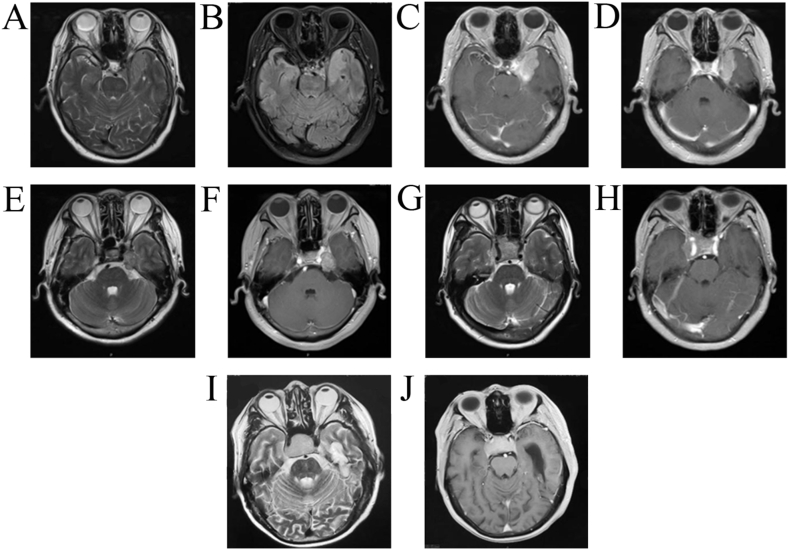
Imaging characteristics of cavernous sinus meningioma (A, B, C, D), schwannoma (E, F) and invasive pituitary adenoma (G, H, I, J). Morphologically, the dural tail sign of cavernous sinus meningioma was obvious with no cavernous sinus swelling and extrusion sign. (A) Heterogenous mild hyperintensity on T2WI. (B) Heterogenous hyperintensity on FLAIR. (C, D) Heterogenous enhancement of enhanced T1. Lesions of schwannoma protrude from the cavernous sinus to the posterior cranial fossa. (E) Heterogenous mild hyperintensity on T2WI. (F) Heterogenous enhancement of enhanced T1 without the dural tail sign of cavernous sinus meningioma or cavernous sinus swelling and extrusion sign. (G, H) Case 1 with heterogenous mild hyperintensity on T2WI and heterogenous enhancement of enhanced T1. (I, J) Case 2 with homogenous mild hyperintensity on T2WI and homogenous enhancement of enhanced T1.
